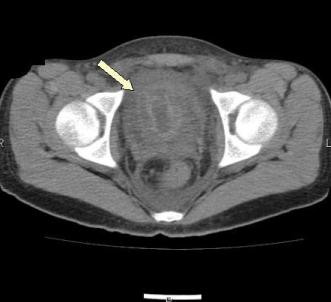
Abdominal CT revealing a marked thickening and irregularity of the bladder wall consistent with bladder hematoma.
